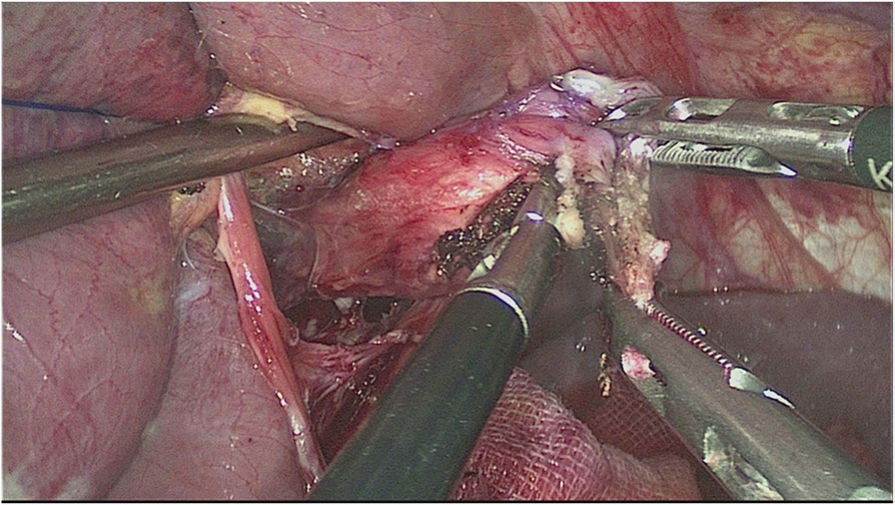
An ultrasonic knife or electric spatula was used to cut the closure line parallel to the esophagus on the right posterior wall of the esophagus’s broken end from the dorsal side to the ventral side to expose the mucosa
